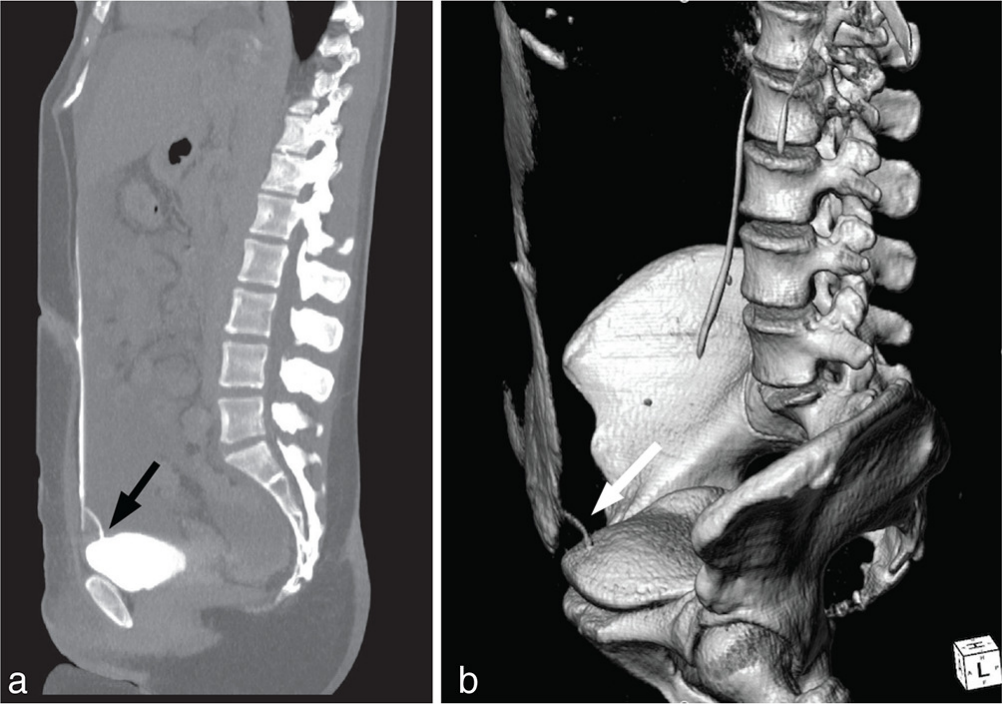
Sagittal CT maximum intensity projection image (a) and volume rendering technique reconstruction (b) both from the ultra-late scan (40 min after the injection of iodinated contrast agent) showing the tearing of contrast agent from the dome of the urinary bladder (arrows).
